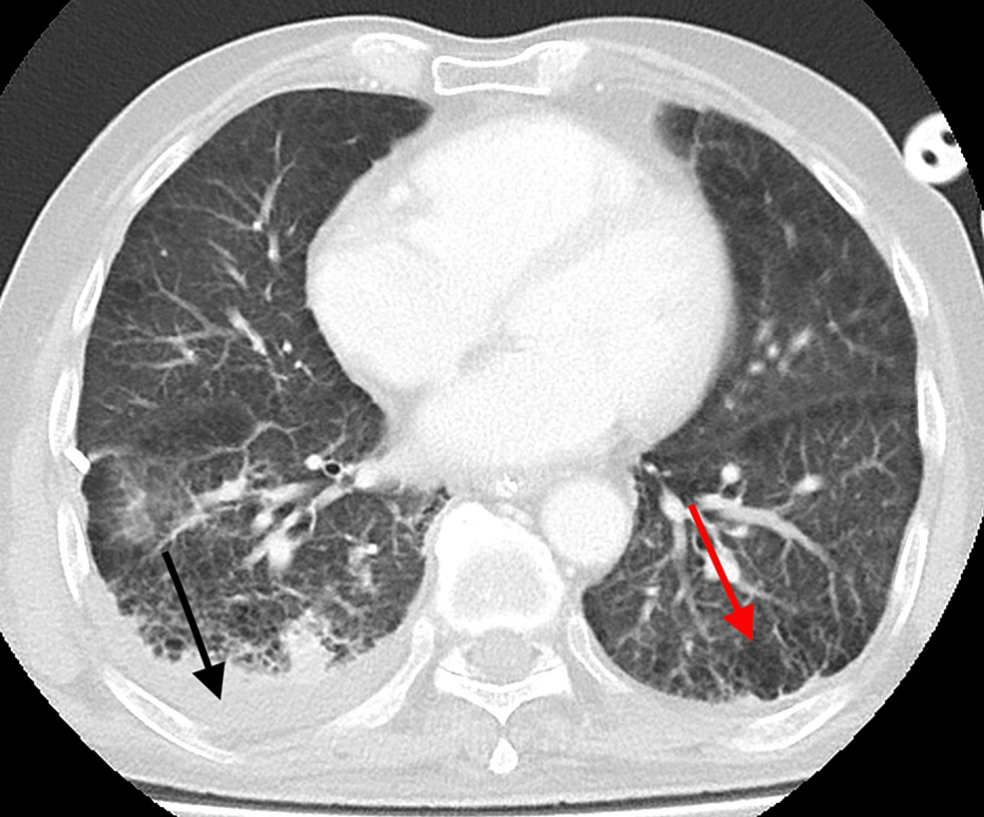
Axial view of the computed tomography of the thorax showing loculated right pleural effusion (black arrow) and emphysematous changes (red arrow)
